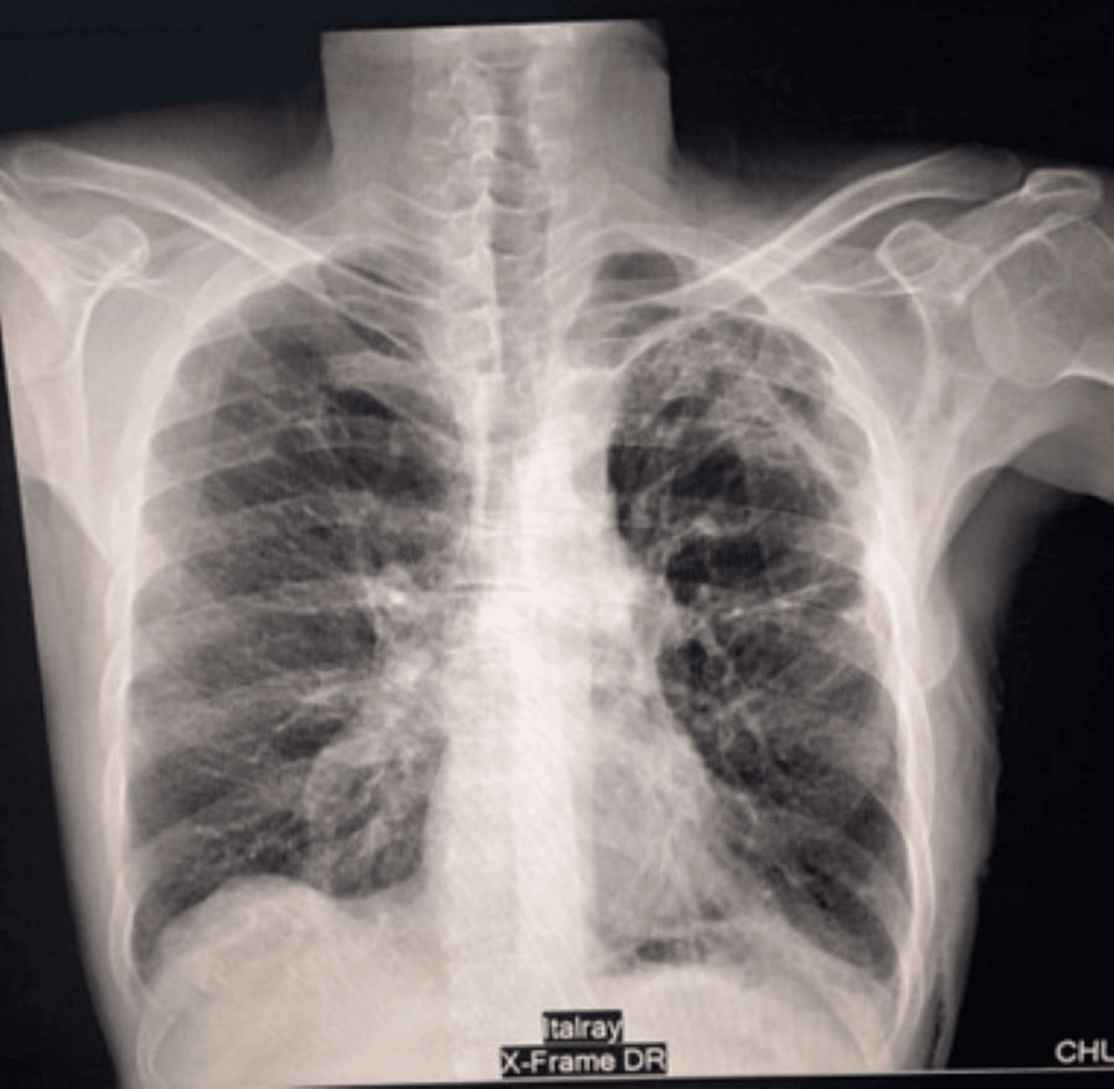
The discharge chest X-ray The patient's discharge chest X-ray was satisfactory
